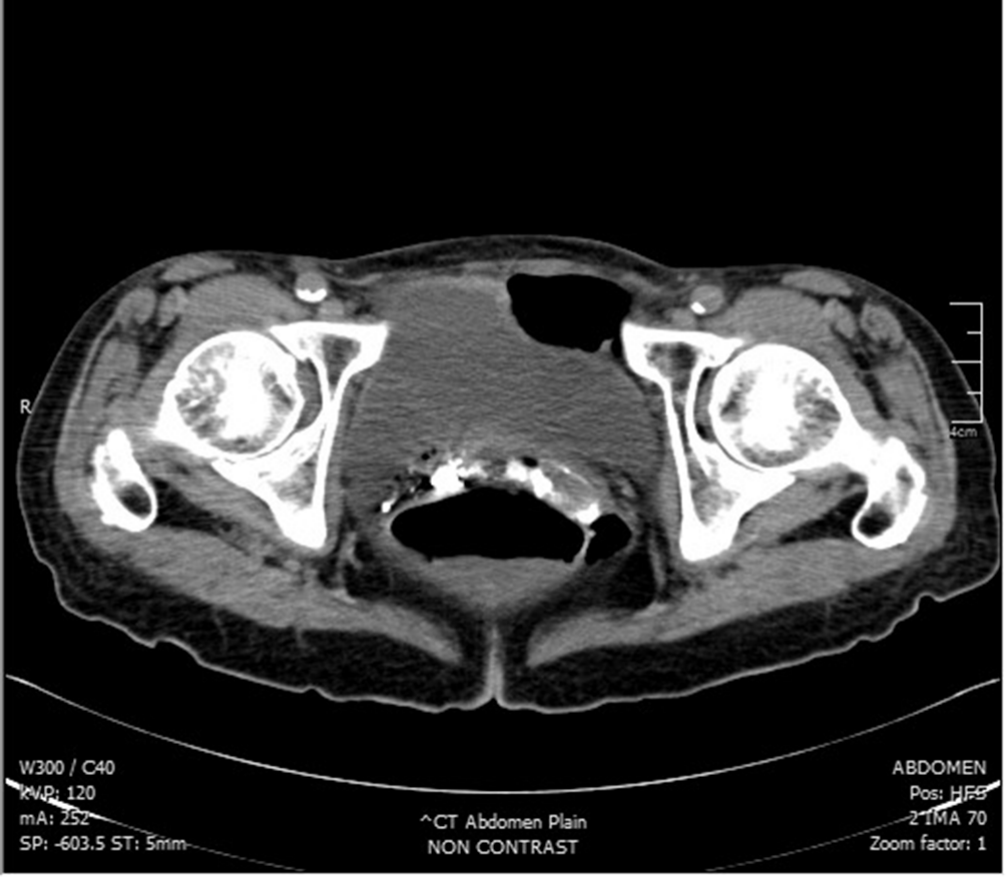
Plain CT showing barium contrast within the uterus and pouch of Douglas. Minimal free fluid and air can also be visualised in the pouch of Douglas.
